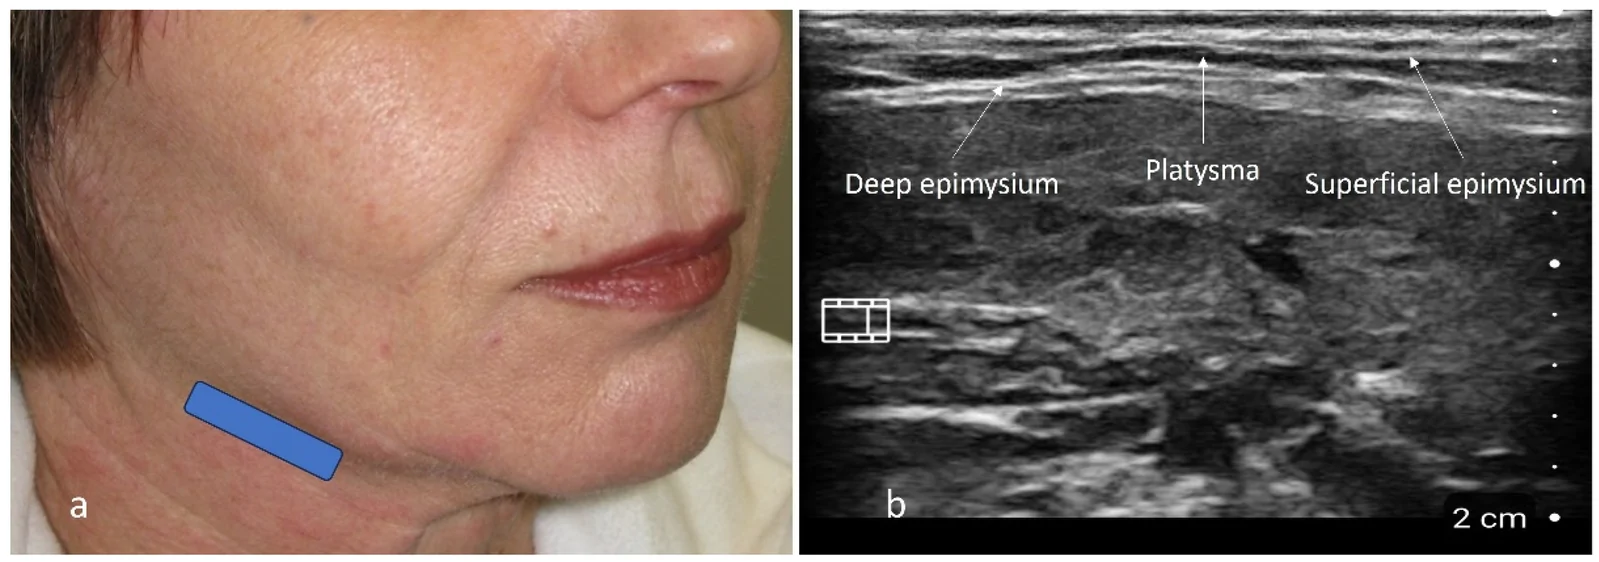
(a) The blue rectangle represents the position of the 20 MHz ultrasound probe. (b) By ultrasound, the platysma muscle is observed as a hypoechoic thin band under the thick hyperechoic epidermis, dermis, and subcutaneous tissue. The PL is enveloped by a superficial and deep epimysium, which appear as a hyperechoic line above and under the hypoechoic PL muscle.
